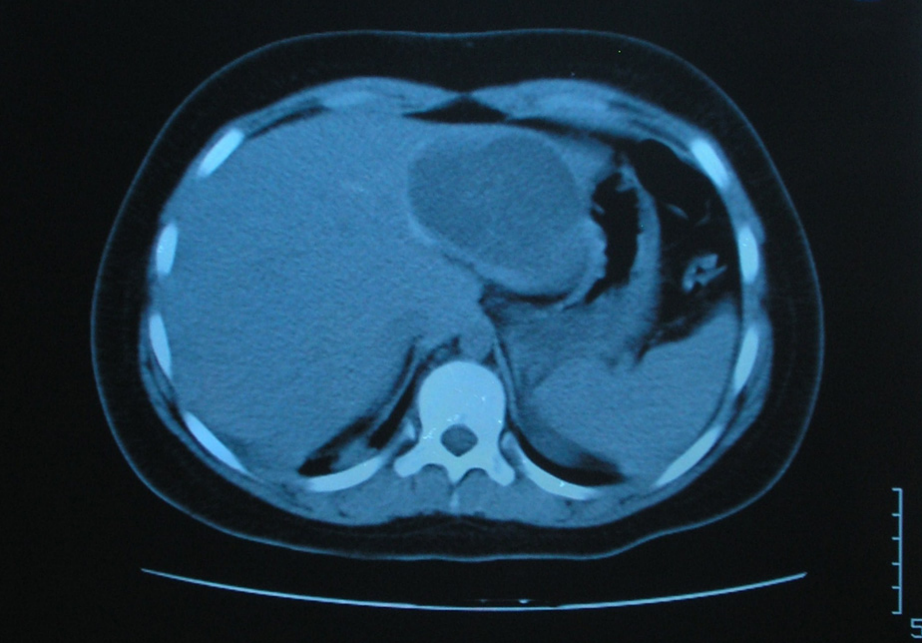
Computed tomography abdominal scan shows a cystic lesion in the left lobe of the liver.
